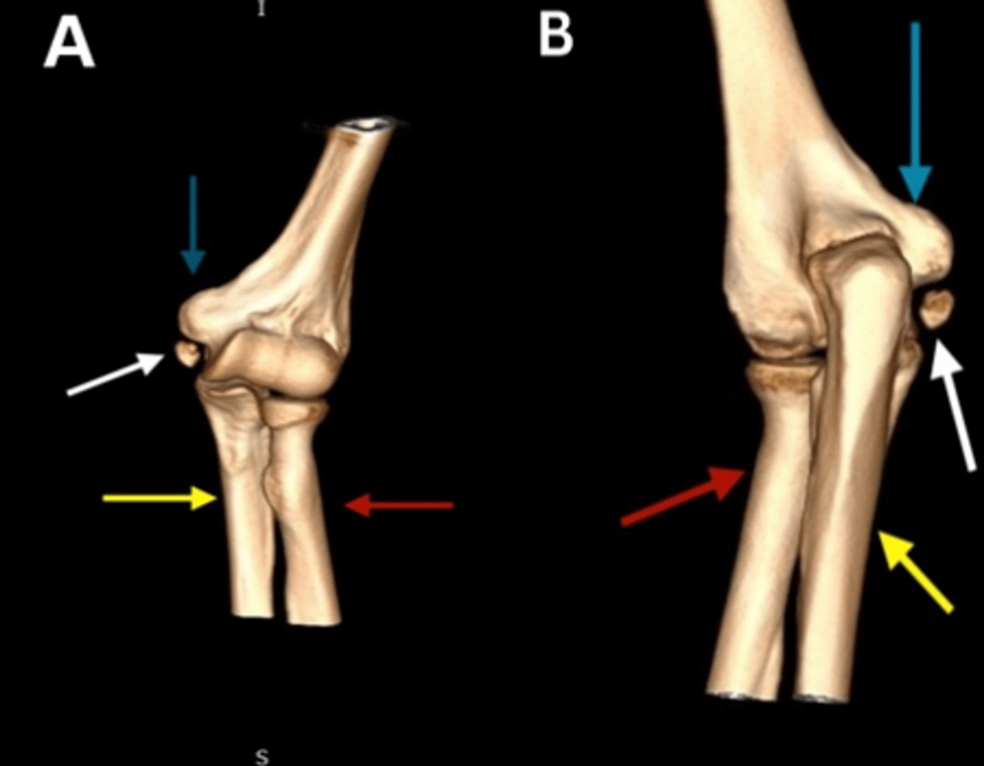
A 3D CT scan reconstructed a model of the left elbow joint (A) anterior view; (B) posterior view The white arrows indicate the os subepicondylare mediale. The blue arrows indicate the medial humeral epicondyle. The red arrows indicate the radius. The yellow arrows indicate the ulna.
